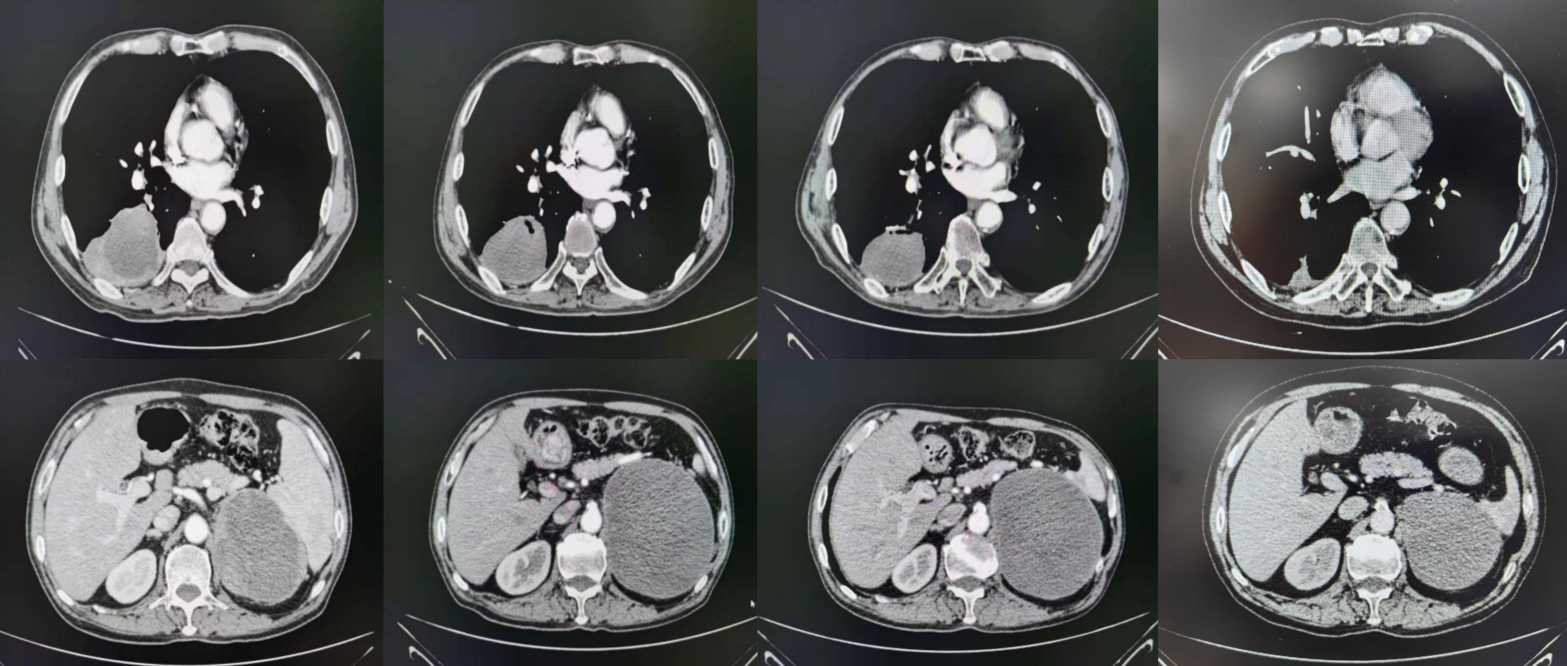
CT scan results of the patient.
